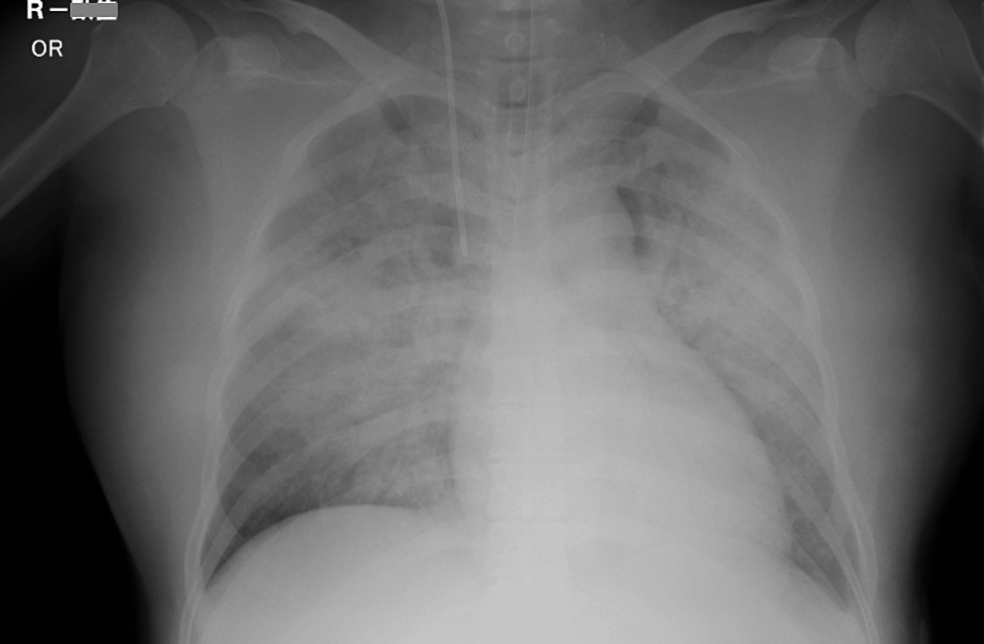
Postoperative chest X-ray shows massive pulmonary edema
